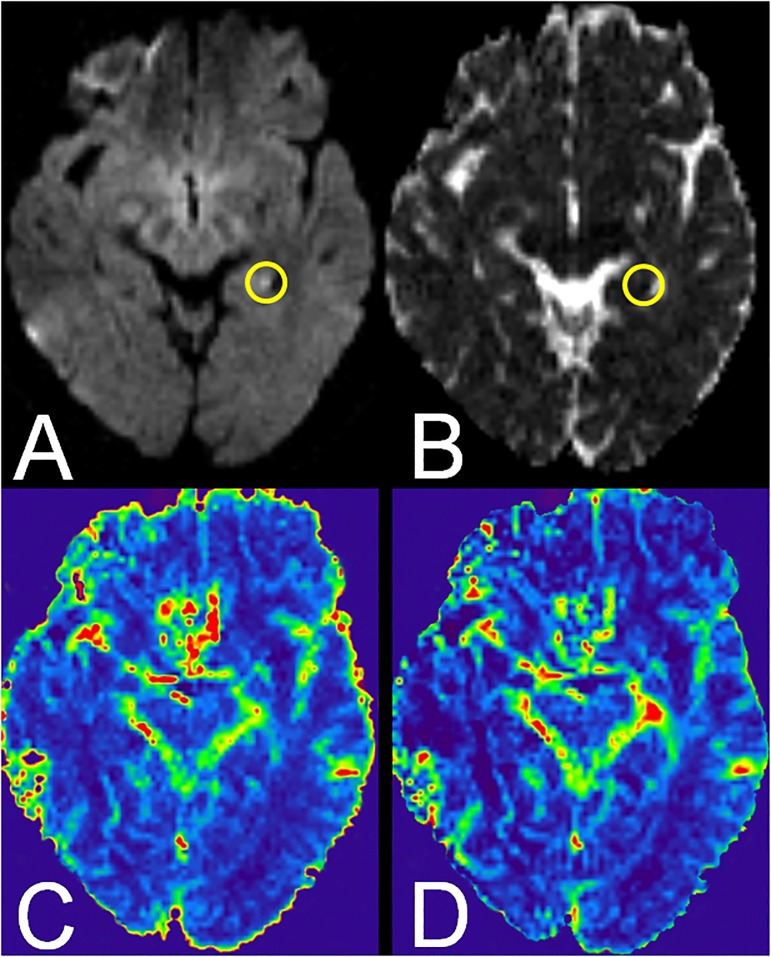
MRI findings in TGA patient 2. A. Focal DWI hyperintensity in the left hippocampus on axial DWI. B. Corresponding decrease of ADC on ADC map. C. CBF and D. CBV are unremarkable.
